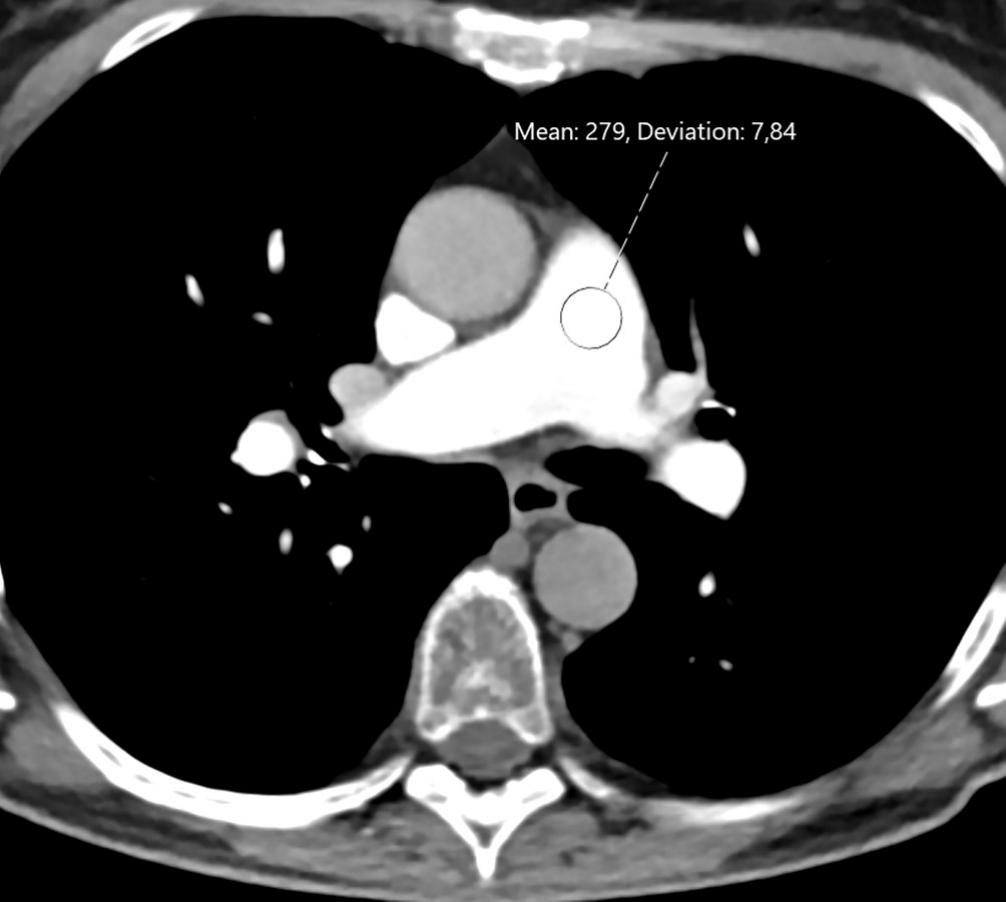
Contrast opacification and image noise in an axial 3 mm slice at the level of the main pulmonary artery in CTPA using 13 ml iohexol 350 mg/ml in a 69-year-old male. The overall image quality was subjectively scored as good–excellent by all readers. CTPA, CT pulmonary angiography.
